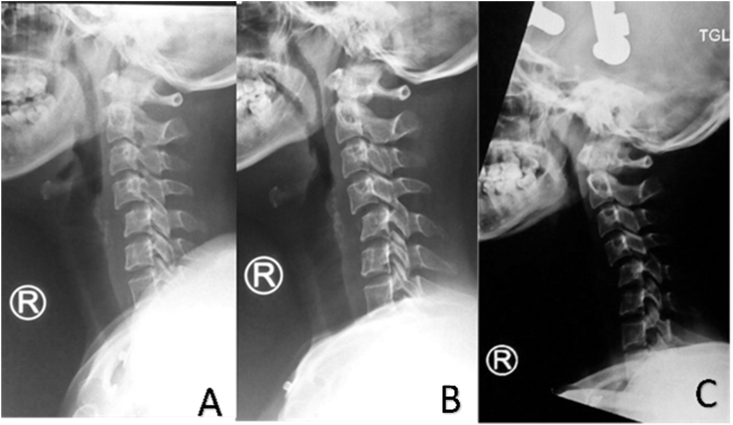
X-Ray of Spine on Gartner Wells Tongs.
